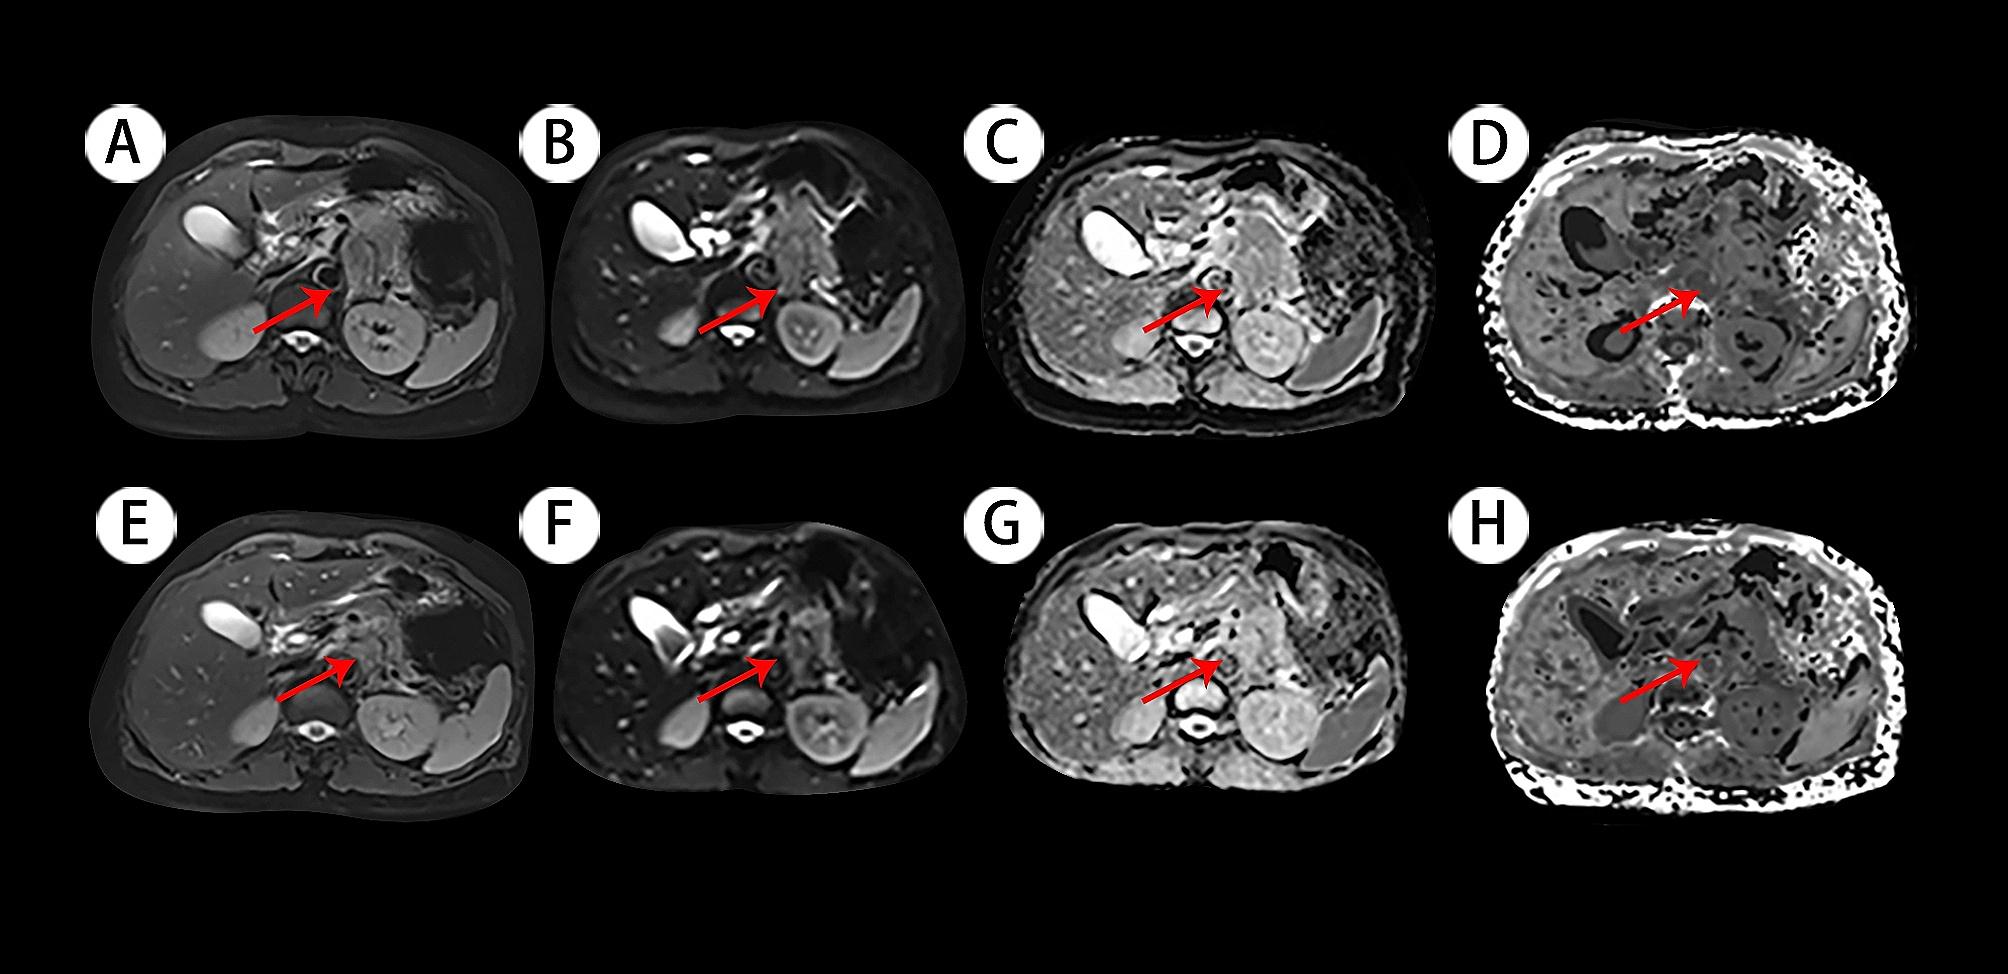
A and E: Axial half-Fourier-acquired single-shot turbo spin echo (HASTE) T2-weighted images; B and F: Diffusion weighted images (DWI) with b-value 0 s/mm^2^; C and G: Apparent diffusion coefficient (ADC) maps; D and H: Diffusion kurtosis image (DKI) maps. Red arrow: the pancreatic lesion. The images in the first row represent the lesion before undergoing chemotherapy, and the images in the second row represent the lesion that underwent two courses of chemotherapy treatment. Before and after chemotherapy, the ADC and MD values increased significantly, while the MK value decreased slightly. The lesion noticeably shrank after treatment, evaluated as PR according to the RECIST criteria. This patient is a 69-year-old woman with a 4.2 × 3.6 cm mass in the tail of the pancreas and liver metastasis. The following parameters were used: ADC_pre_ (1.06 × 10^− 3^ mm^2^/s), ADC_post_ (1.23 × 10^− 3^ mm^2^/s), ADC_change_ (0.16); MK_pre_ (0.61), MK_post_ (0.60), MK_change_ (-0.01); MD_pre_ (1.61 × 10^− 3^ mm^2^/s), MD_post_ (2.58 × 10^− 3^ mm^2^/s), MD_change_ (0.60)
